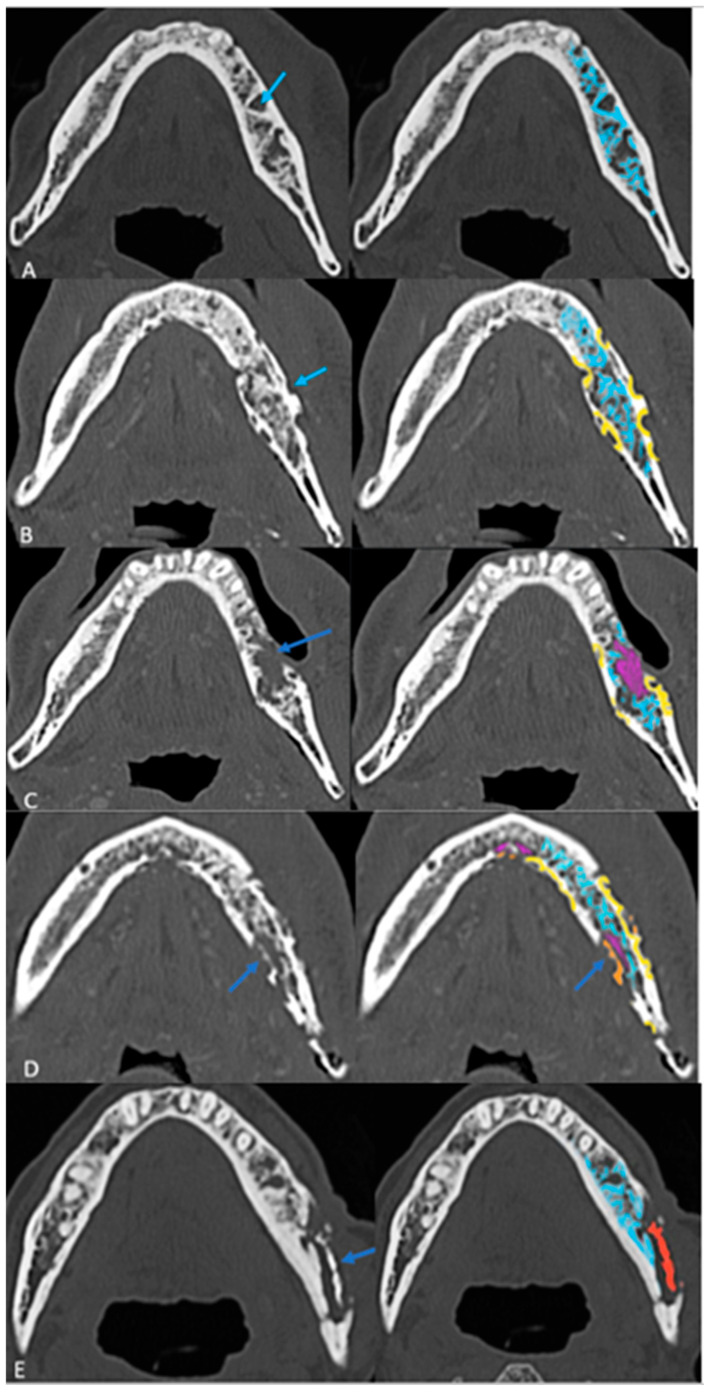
The proposed structured grading system of ORN on axial CT images: (A) Grade 0: Resorption (Early Stage) with thickened and sparse trabecular (blue). (B) Grade 1: Partial thickness cortical resorptions (yellow). (C) Grade 2: Full-thickness cortical resorption with medullary extension (pink areas). (D) Grade 3: Fragmentation/fracture (Orange). (E) Grade 4: Sequestrum (red) (End Stage).
